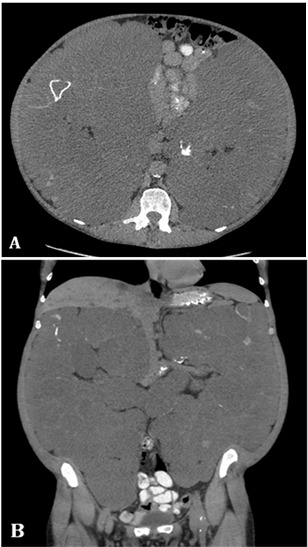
Pre-operative CT scan demonstrating significantly enlarged polycystic kidneys removed using hand-assisted laparoscopic techniques. A. axial view. B. coronal view
